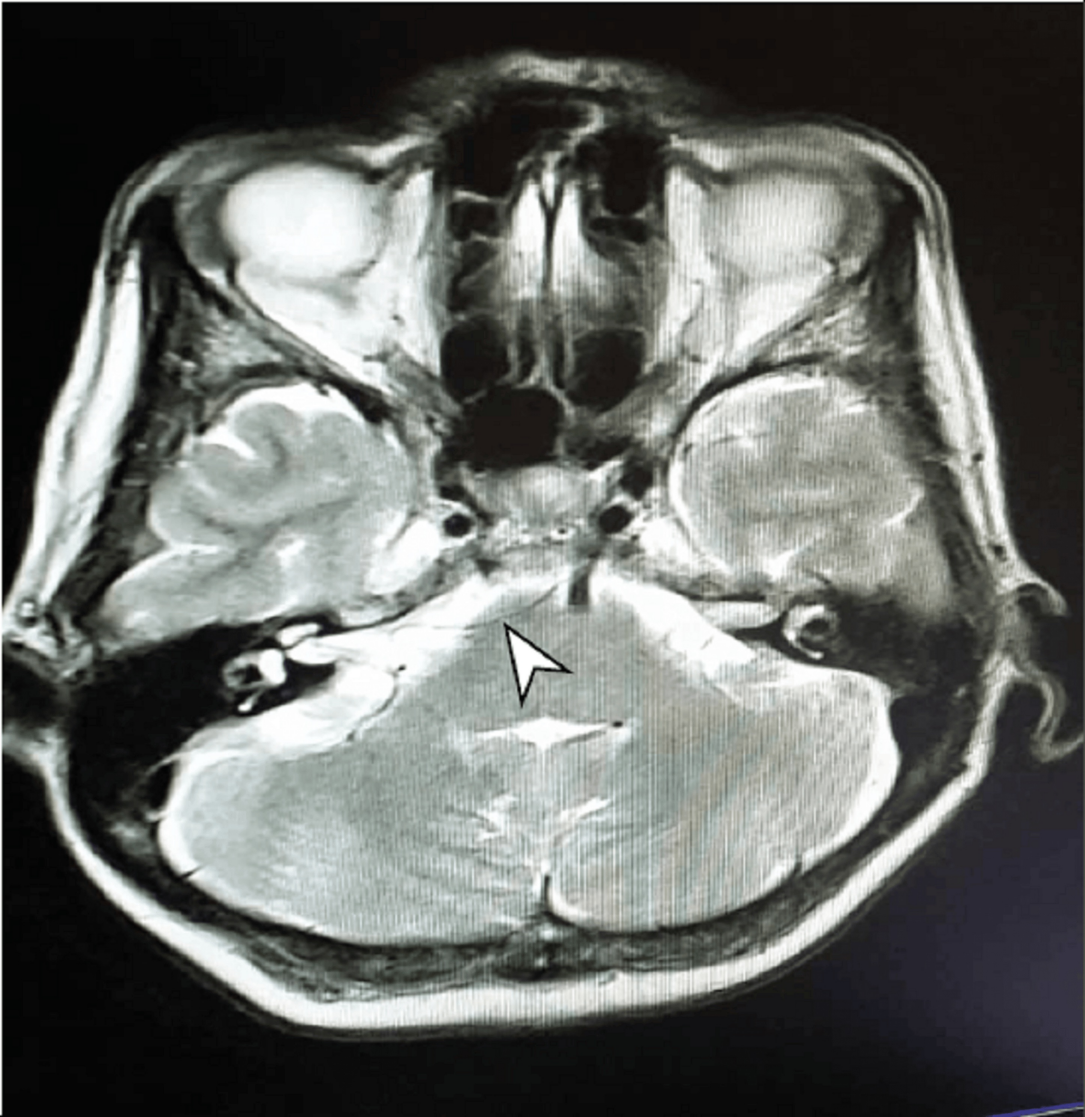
The vascular loop is observed on the T2 axial view magnetic resonance image (indicated by the arrowhead)
